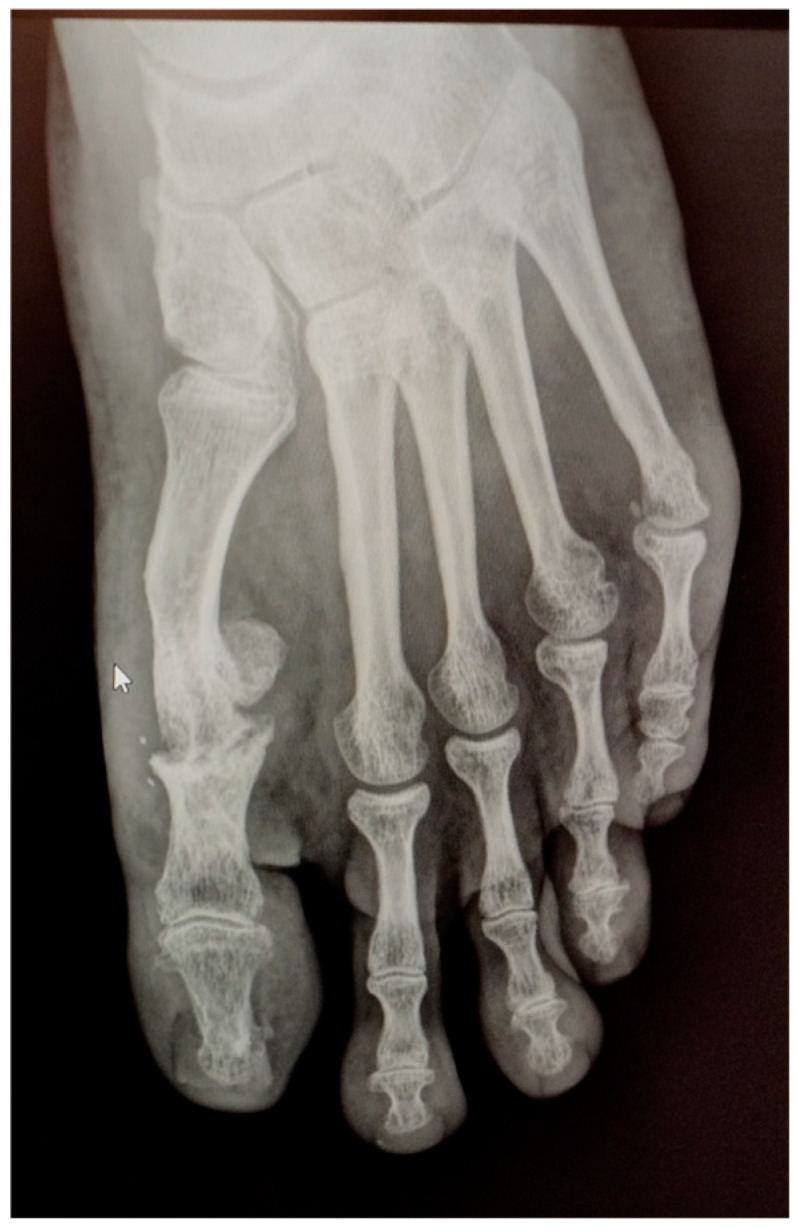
Postoperative radiography one year after the second procedure. Signs of severe joint destruction compatible with resection arthroplasty can be observed.
